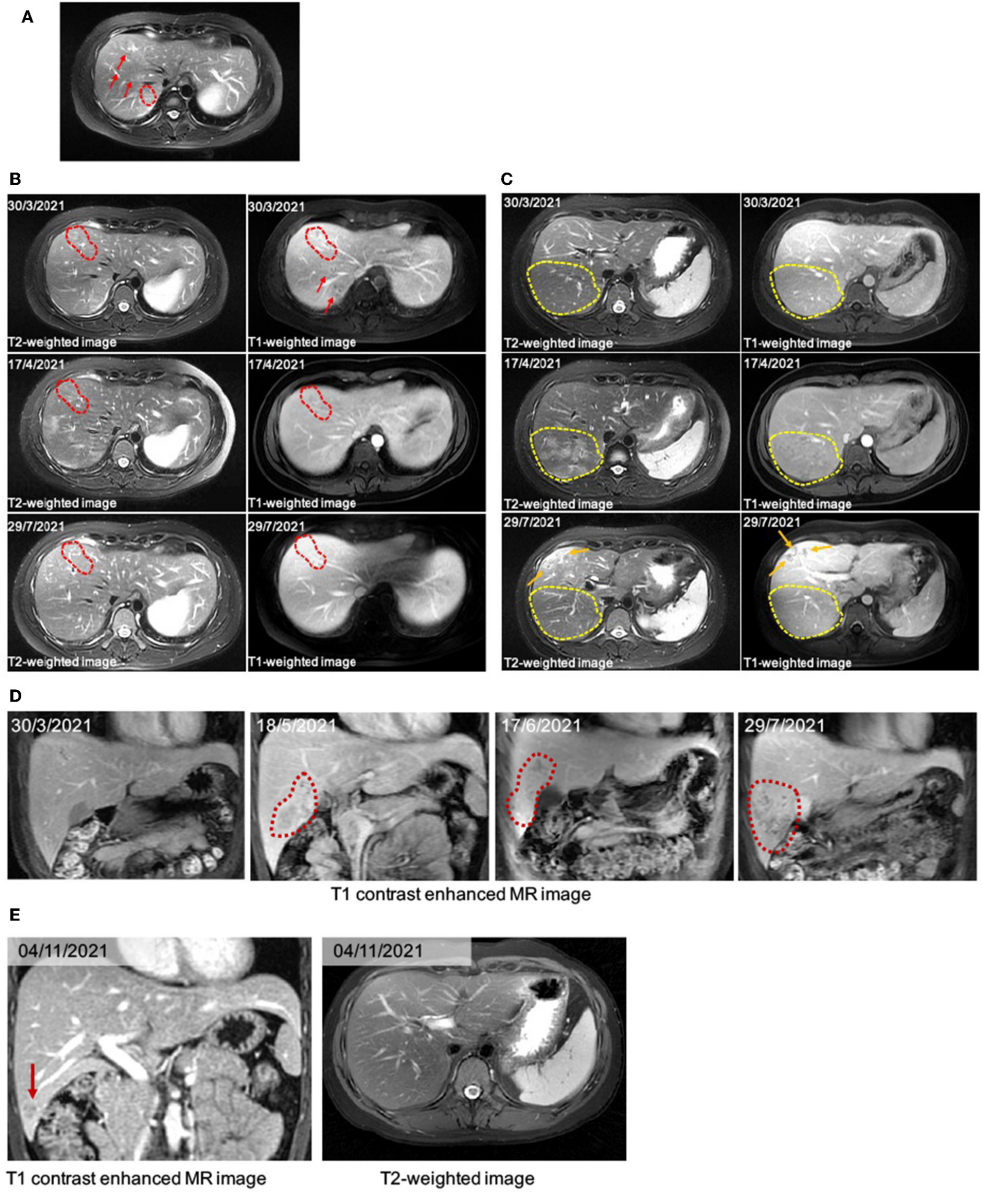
(A) The liver MRI image. There are several typical curved tunnel signs (red arrows and dotted frame) on T2WI. (B) A serial image of the patient before and after anti-helminthic therapy in the hepatic anterior lobe. The image on March 30^th^, 2021, showed typical “curved tunnel” signs in hepatic right anterior and posterior lobe on T1WI and T2WI image (red dotted frames and arrows). On April 17^th^, a month after the first therapy, the area of curved tunnel signs in hepatic anterior lobe decreased. After the following anti-helminthic treatments, the liver MRI image showed further decreased signs (29/7/2021). (C) A serial image showed dynastic change in the hepatic posterior lobe. On the image of March 30th, there were several curved tunnels in the marked yellow dotted frame. The new massive patchy slight hyperintense signals on April 17^th^ were almost gone on the image of July 29^th^. Besides, we observed newly tunnel changes located under the subcapsular (orange arrows). (D) Coronal image showed subcapsular lesion appeared first on the image of May 18^th^, 2021. During the anti-helminthic therapy course, the lesion expanded and was progressed into abscess. (E) On the T1 contrast enhanced MR image, the subcapsular lesion in hepatic right lobe decreased significantly (red arrows).
